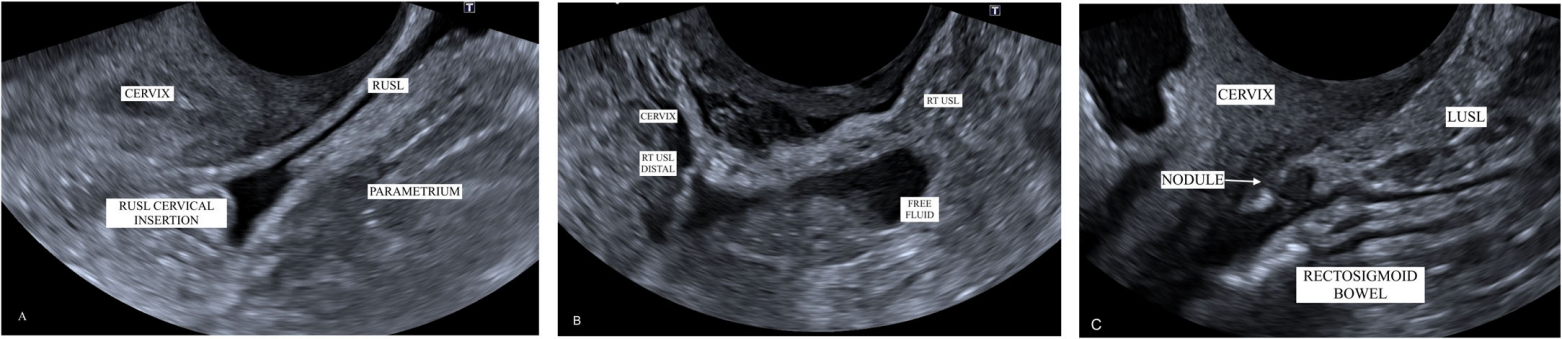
TVS ultrasound images of the USL. (a) a normal USL with a thin, hyperechoic, homogeneous, smooth structure with adjacent physiological fluid in a longitudinal plane. (b) USL with endometriotic involvement in a patient where the USL is highlighted with free fluid and seen to be thickened and heterogeneous with an irregular contour in a patient with endometriotic bowel lesions and background adenomyosis. (c) A hypoechoic endometriotic nodule within the USL at the cervical insertion. TVS, transvaginal ultrasound; USL, uterosacral ligaments.
